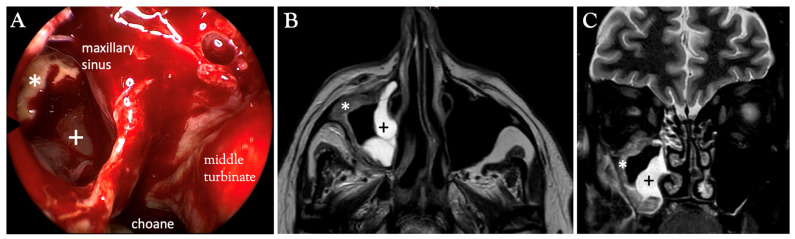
54-year-old male confirmed with right AIFR (*Mucor*). (A) Intraoperative endoscopic view after opening the right maxillary sinus. Within it, congested yet still vital sinus mucosa (+) is clearly distinguishable from the whitish necrotic sinus mucosa (asterisk) as indicated by the corresponding MRI ((B) axial, (C) coronal).
